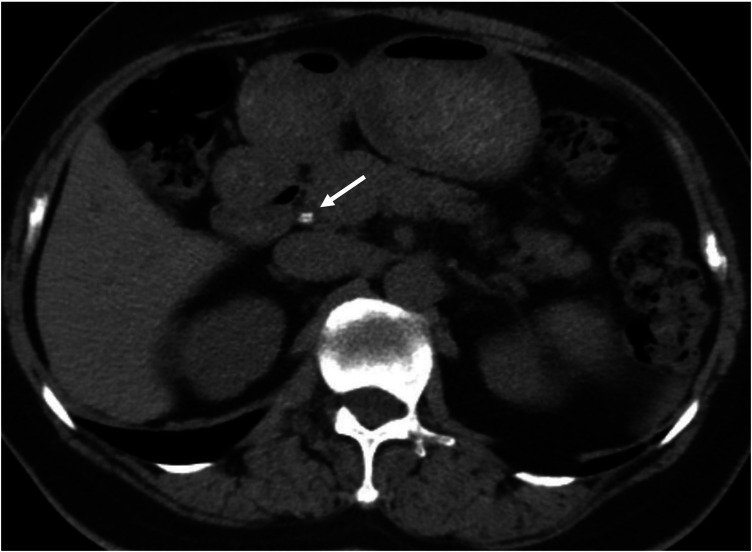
Axial view of the non-contrast abdominal CT scan on admission. The white arrow indicated the radiopaque stones in the common bile duct. No pneumobilia was observed in the intrahepatic or extrahepatic bile ducts.
